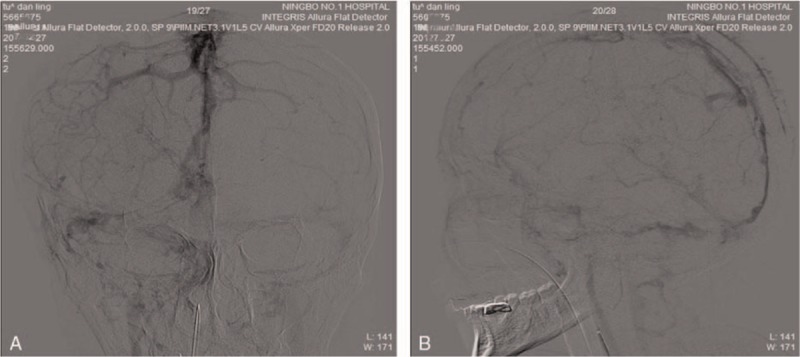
The results of DSA of 2 weeks after endovascular treatment. A. Posteroanterior radiograph; B. Lateral imagine. DSA = digital subtraction angiography.
